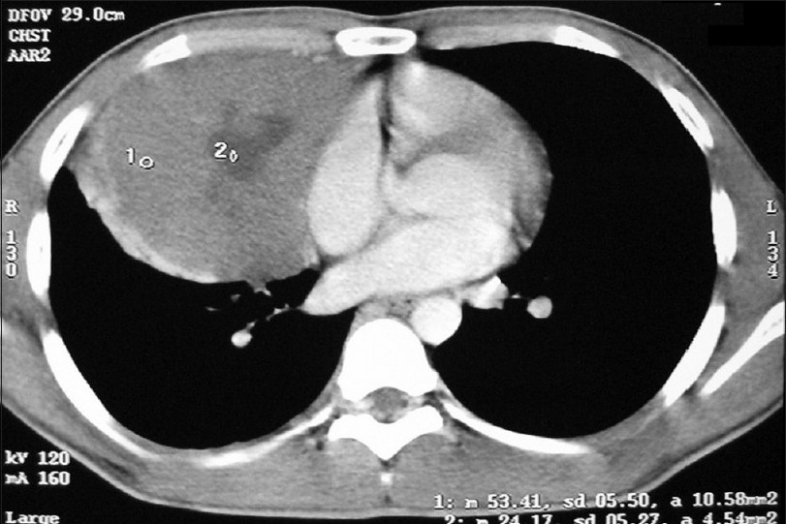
CT chest (2006 ) shows anterior mediastinal mass extending into right side of chest
